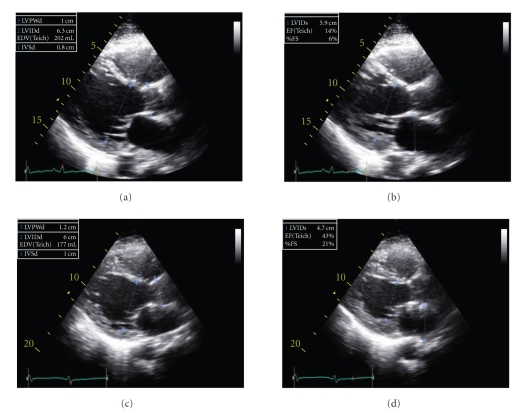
Parasternal long-axis views showing severe baseline LV dysfunction (Figures 4(a) and 4(b)) and improved ejection fraction on the followup study (Figures 4(c) and 4(d)).
